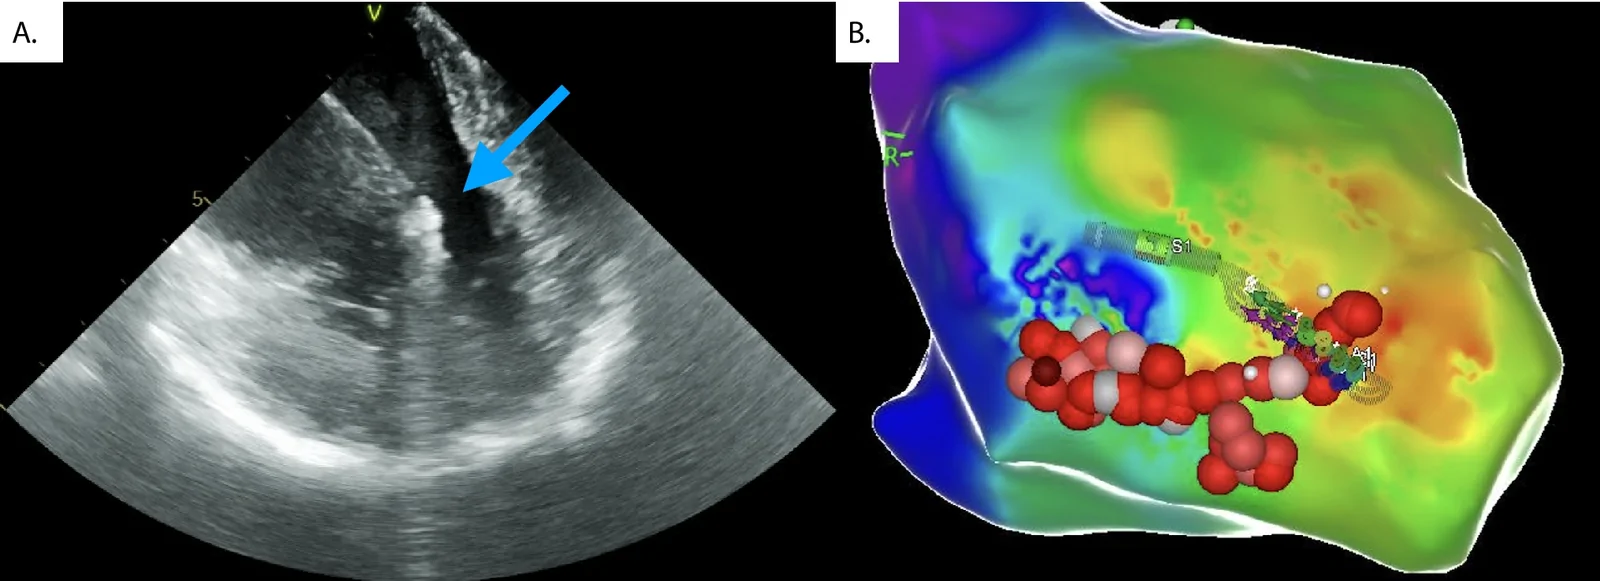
A. Intracardiac echocardiography shows RF catheter on head of papillary muscle which correlated to B. Successful ablation site of papillary PVC mediated VT
